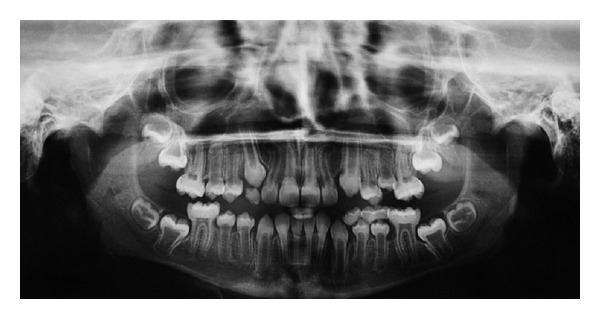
Panoramic radiography showing large pulp chambers in all teeth, and absence of agenesis, impaction, or supernumerary roots.
